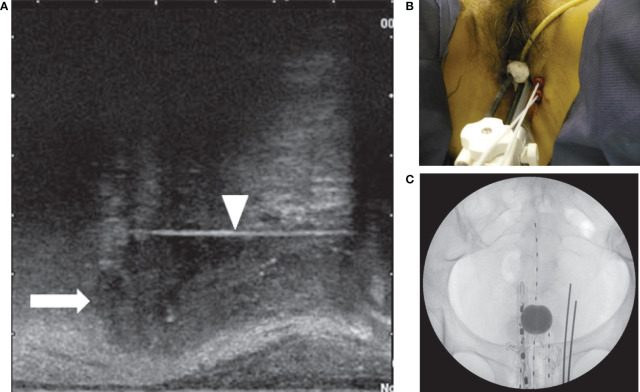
(A) Sagittal view of the transrectal ultrasound showing the parametrial invasion (arrow) and the interstitial perineal applicator (arrowhead). The applicator is inserted up to the cranial margin of the parametrial invasion. (B) Two perineal interstitial applicators were inserted to the left of intracavitary applicators. (C) Anterior x-ray of intracavitary and interstitial applicators. Also seen is a rectal dosimeter.
